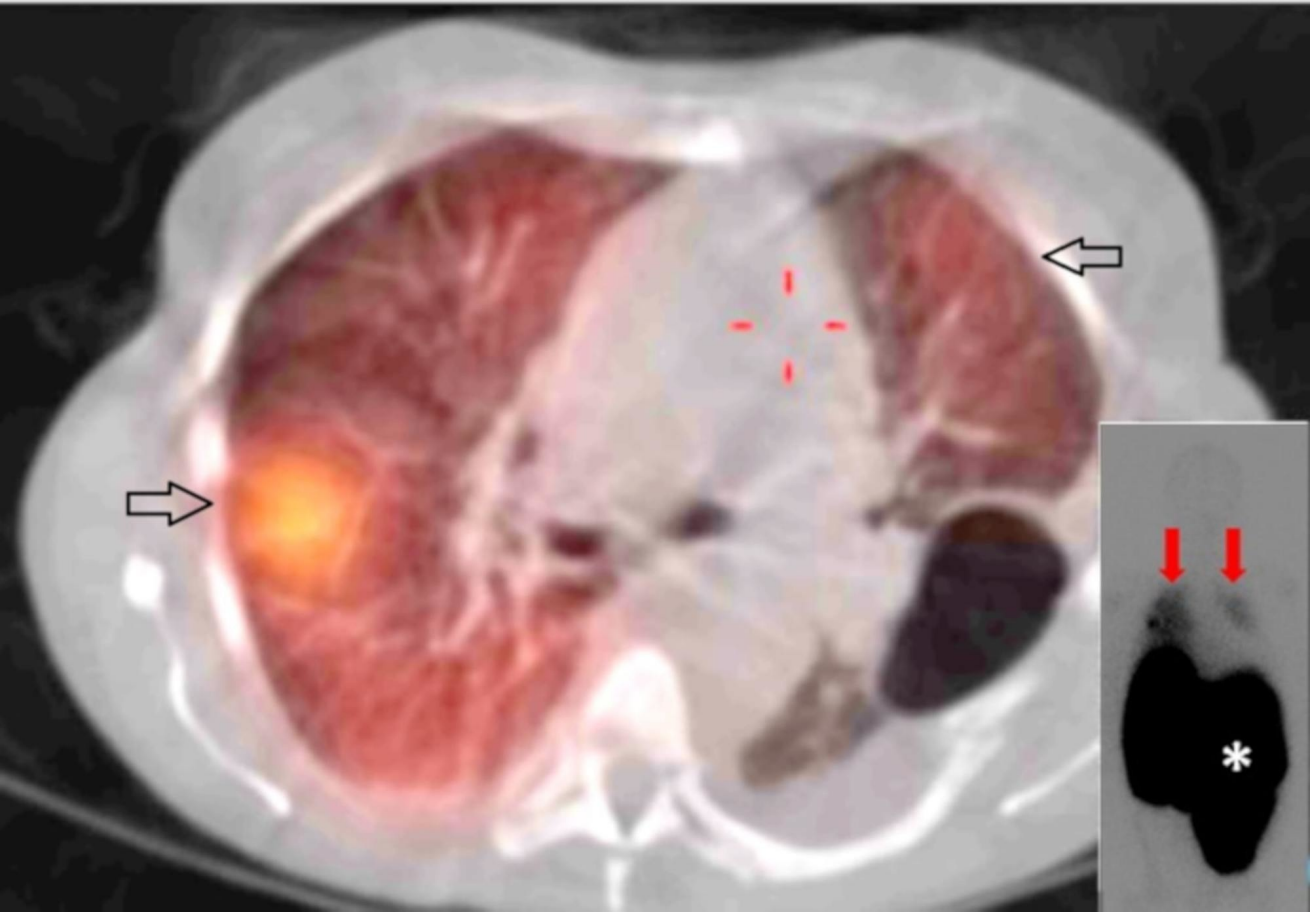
Increased uptake on SPECT/CT Technetium-99m sulfur (Tc-99m sulfur) colloid single photon emission computed tomography/CT (SPECT/CT) axial fusion image showing increased uptake in the thorax (black arrows). Planar scintigraphy images (inset lower right) likewise demonstrate increased uptake in both the right and left hemithorax (red arrows) while highlighting the massive splenomegaly characteristic of myelofibrosis (asterisk)
